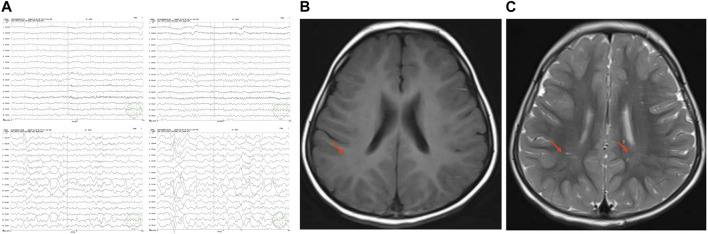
EEG and cranial MRI. (A). EEG of the patient at age 7 months. Abnormal background rhythm was found. (B–C). Axial T1MI and T2MI on Cranial MRI. Symmetric patchy abnormal signals in the bilateral parietal white matter and adjacent to the bilateral lateral ventricles, and insufficient cerebral white matter myelination were observed. The nuclei in the bilateral basal ganglia showed symmetric slightly short T1 and slightly short T2 signals; the bilateral lateral paraventricular region and bilateral parietal white matter revealed symmetric patchy slightly long T1 and slightly long T2 signals, isointense signals on fluid attenuated inversion recovery (FLAIR) image, and slightly low-intense signal with indistinct edges on diffusion weighted imaging (DWI).
